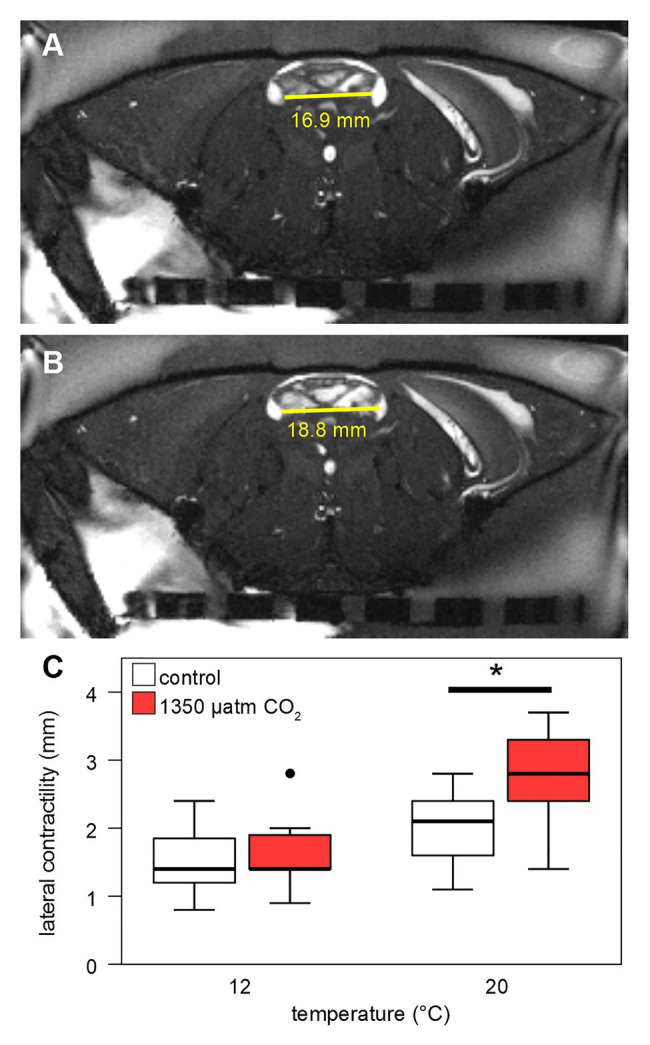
Contractility of the heart. Contractility was calculated from CINE MRI as difference between end-systolic (A) and end-diastolic (B) lateral diameter of the heart, in an axial slice including the two lateral ostia (endpoints of the horizontal yellow line). (C) Lateral contractility of the heart (in mm) is shown for 12 and 20°C control and high CO_2_-levels. Whisker = 1.5 × interquartile range (IQR), box = IQR, bold line = median, and circle = outlying data point. Hypercapnia caused a significant increase in contractility at 20°C (Student’s *t*-test, *p* < 0.05).
